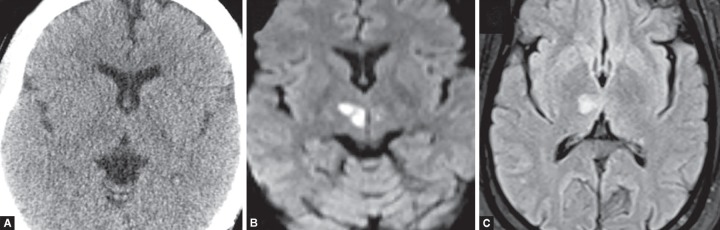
Acute right thalamic infarction not appreciated on CT. (A) but very well on MRI. It shows (B) diffusion restriction on DWI and (C) hyperintensity on FLAIR in the affected right thalamus
